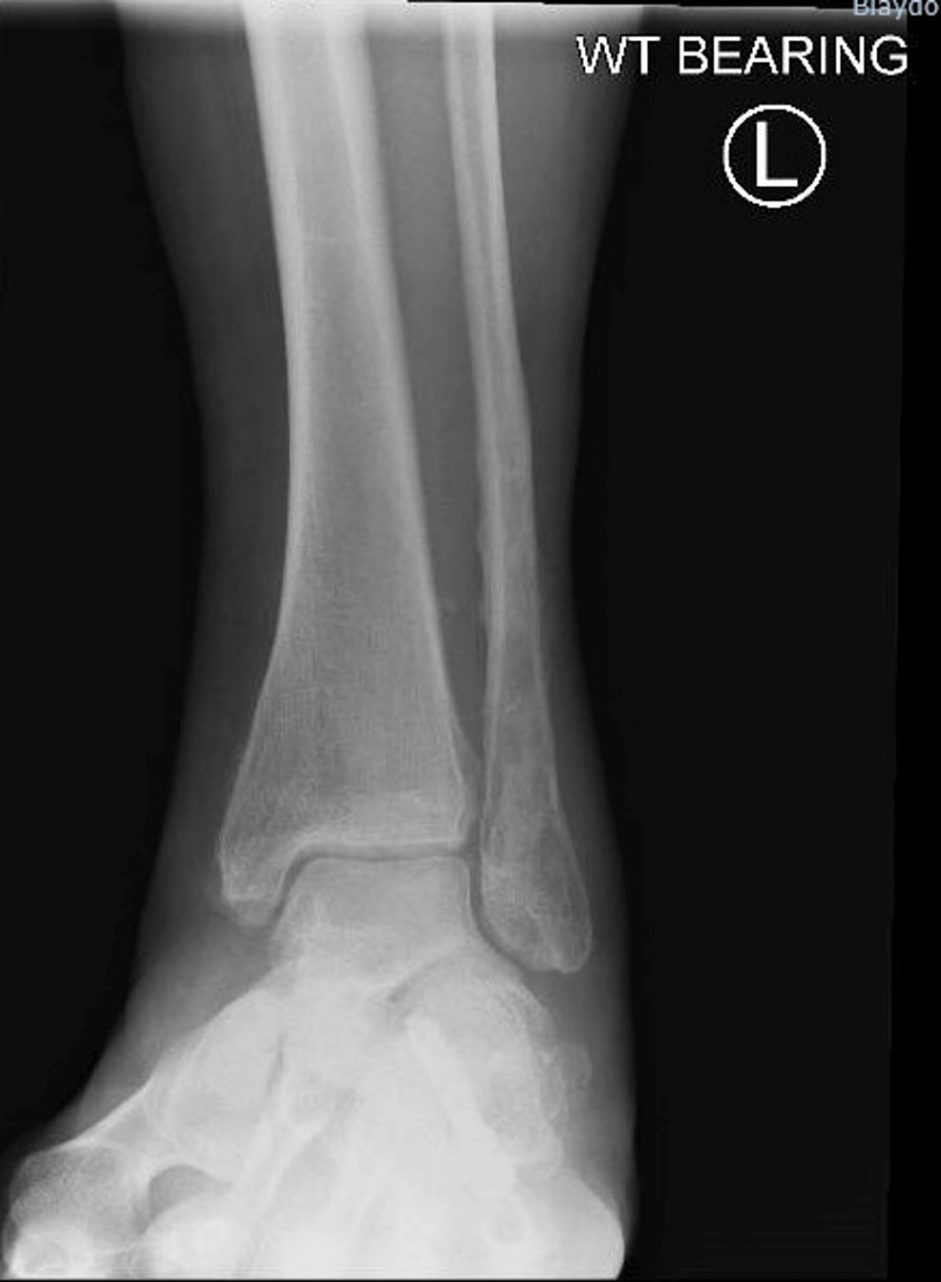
Final AP radiograph showing no bony tunnel around medial malleolus and reconstruction of arch of the foot AP: anteroposterior
